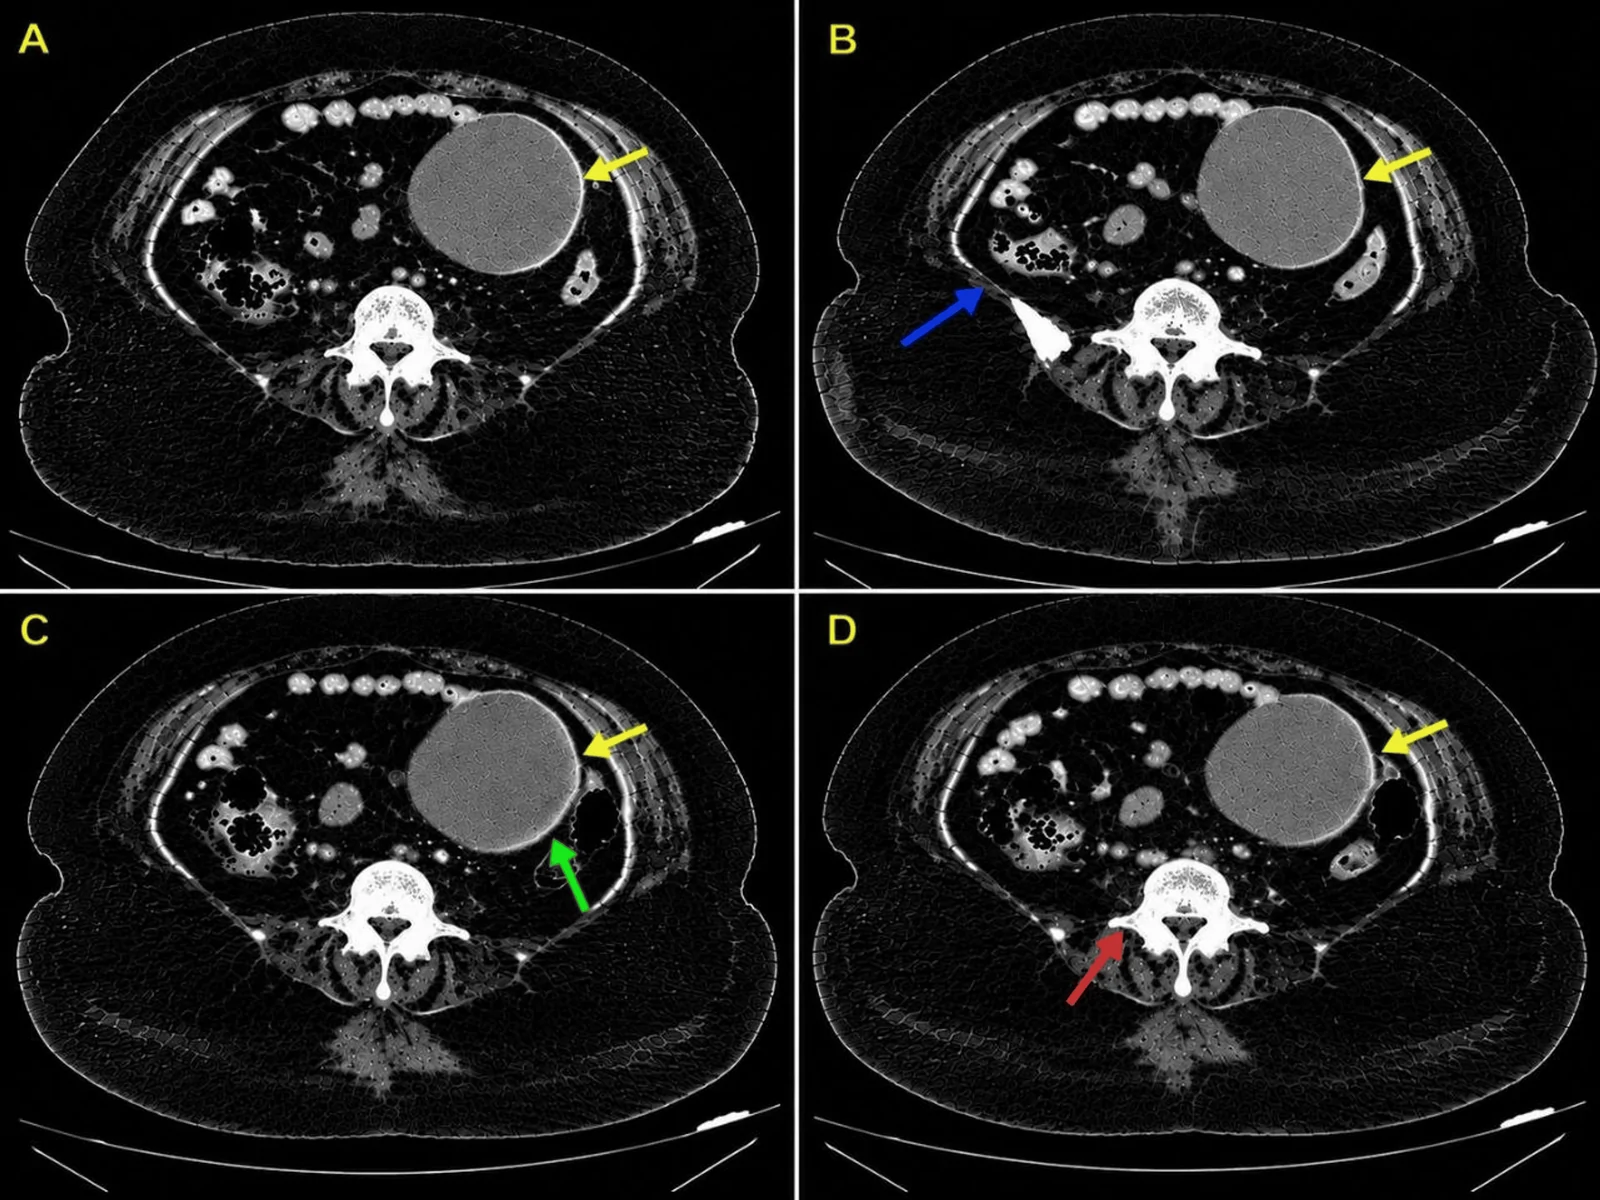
Contrast-enhanced computed tomography (CT) of the abdomen and pelvis. Axial images (A–D) obtained at sequential levels demonstrate a well-circumscribed, predominantly cystic, left adnexal mass measuring approximately 6.0 × 10.0 × 10.5 cm (yellow arrows), arising from the left ovary. The lesion is unilocular with a thin, smooth wall (green arrow) and homogeneous low-attenuation fluid content. There are no internal septations, mural nodules, or solid enhancing components. Mild mass effect on adjacent bowel loops is noted (blue arrow), without evidence of adjacent organ invasion, ascites, or enlarged lymph nodes (red arrow).
